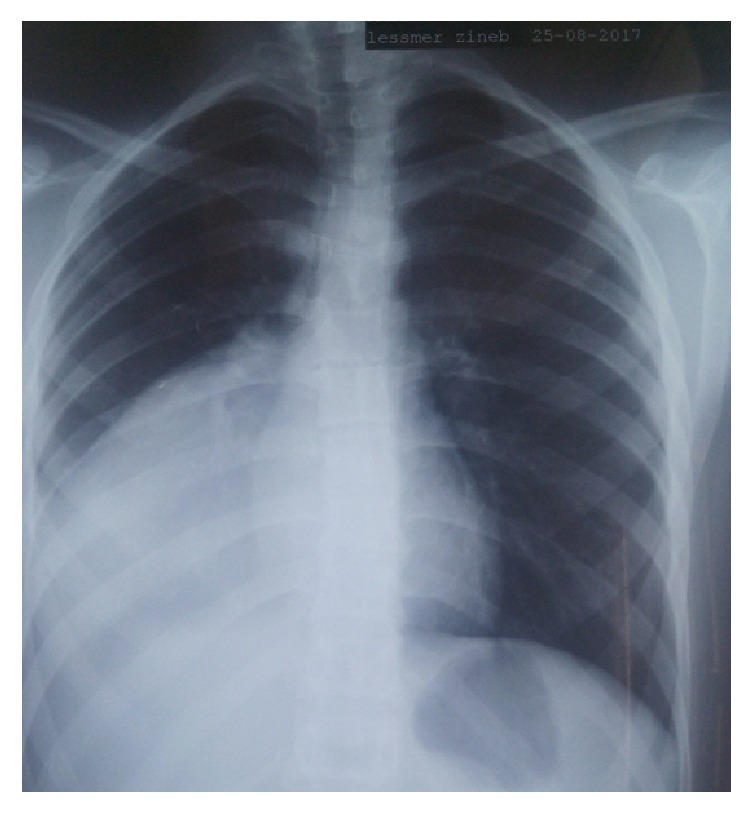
Posteroanterior chest roentgenogram showing a right basal opacity of watery tonality with convex upper limit (patient 1).
